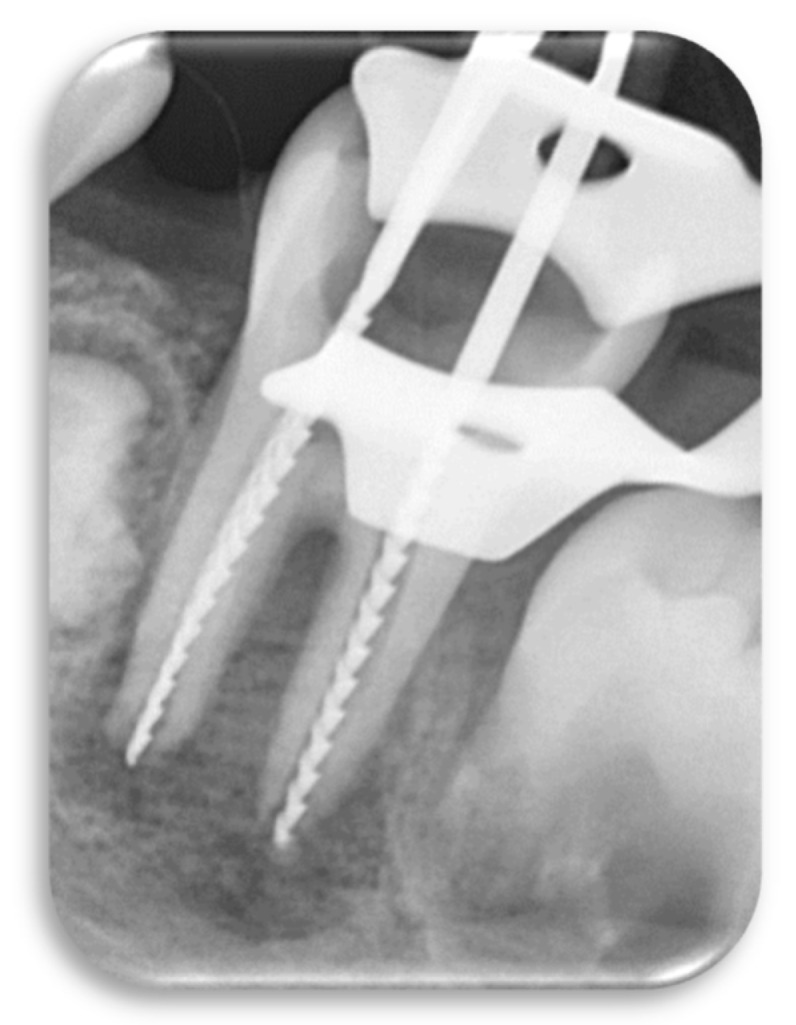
Determining the working length radiographically.
